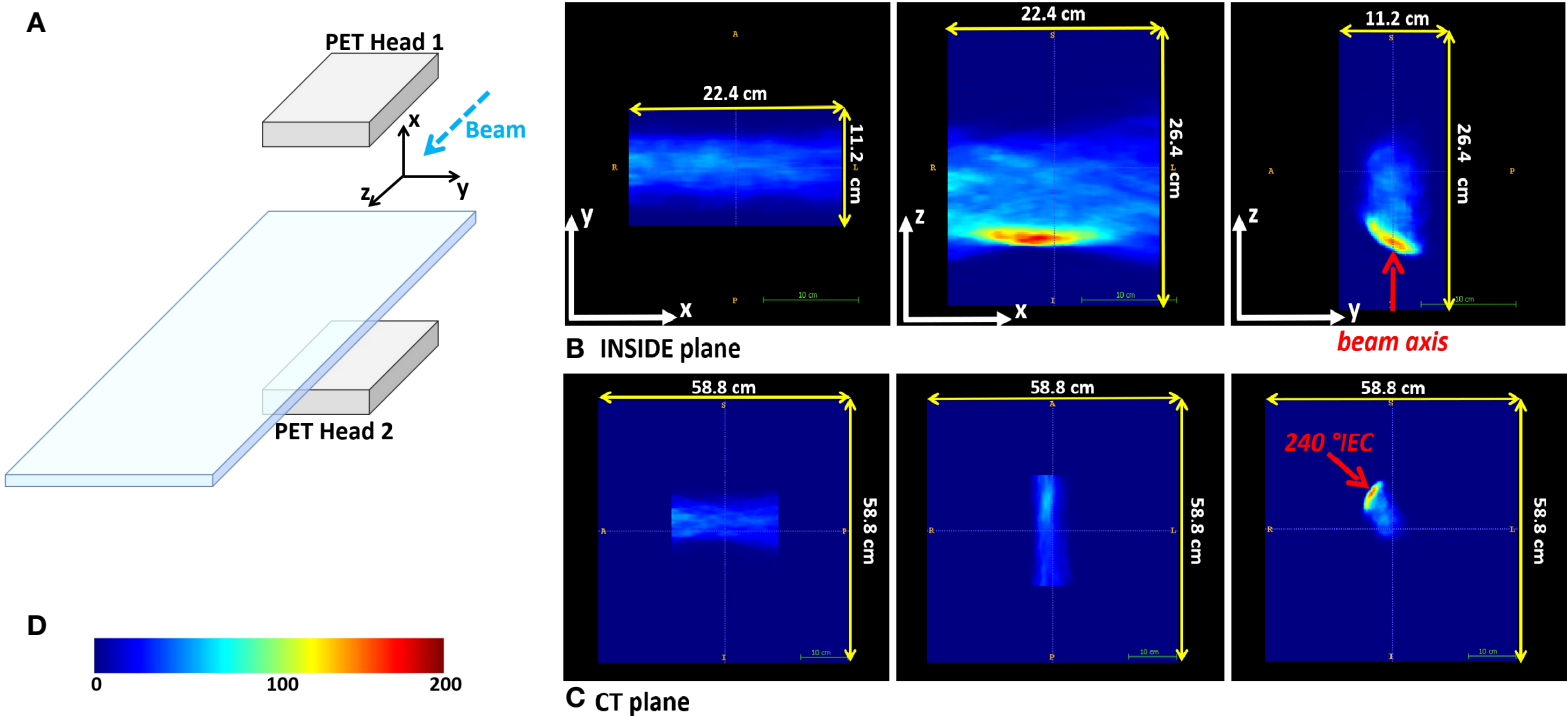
Reference frames. (A) Sketch of the INSIDE setup and reference frame. (B) Example of the three orthogonal projections of a reconstructed PET image for patient 002P (see 2.3). (C) The same reconstructed PET image in the CT reference frame. In (B, C) the beam axis is indicated with the red arrow. (D) The color-scale of the PET images.
